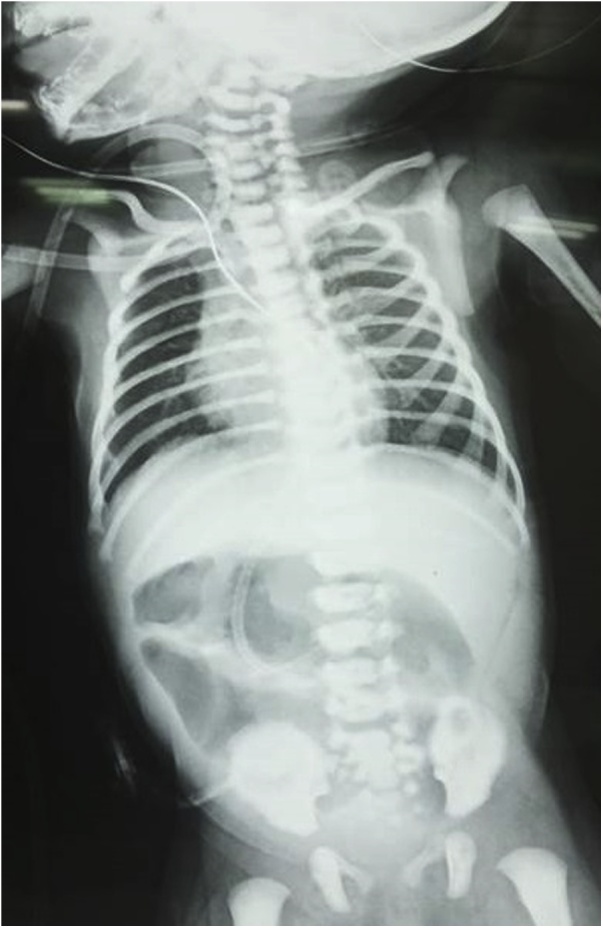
X-ray abdomen showing few dilated bowel loops, paucity of distal gas shadows and nasogastric tube in the right upper quadrant of abdomen.
